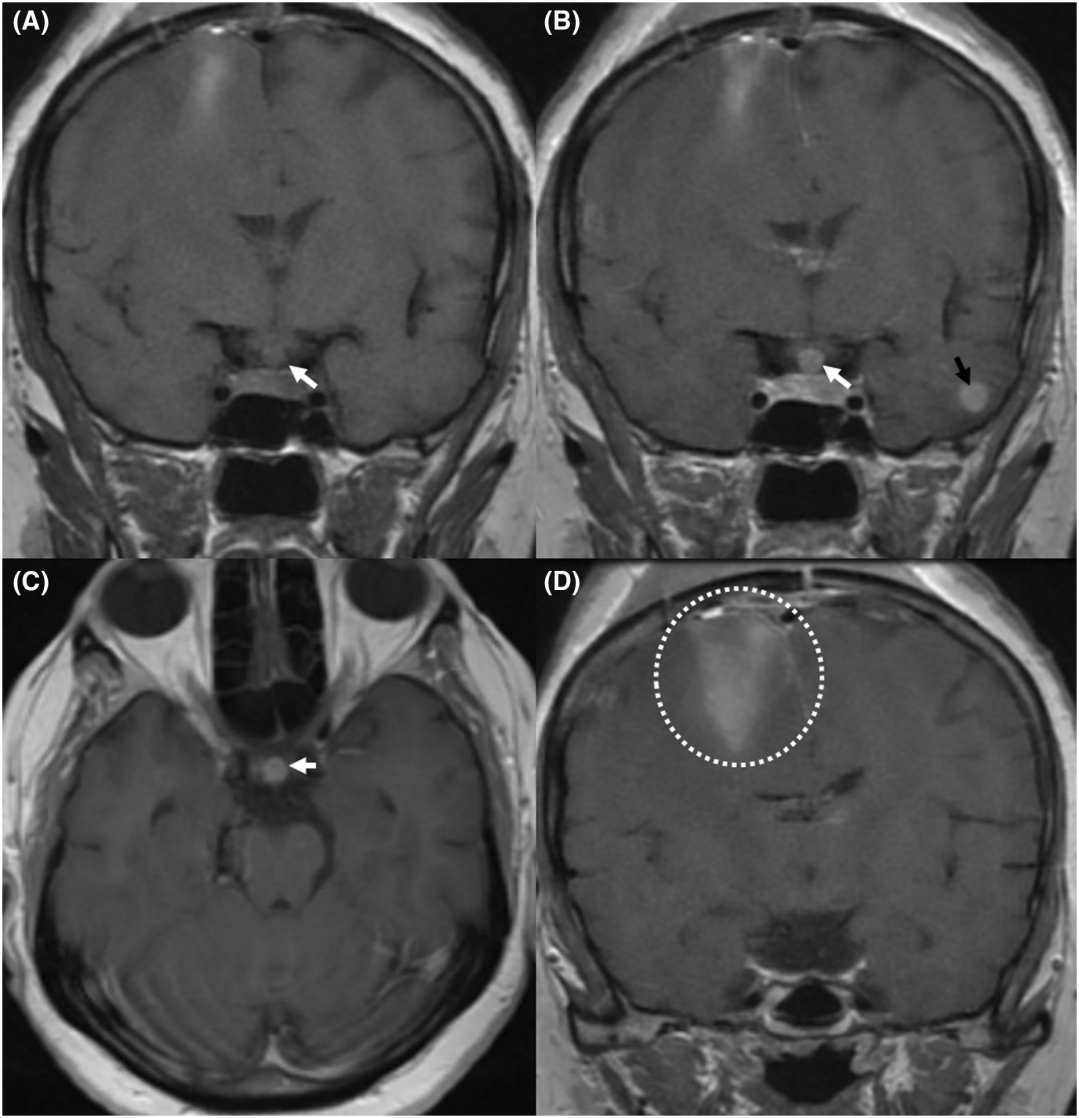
Postoperative MRI screening shows a new lesion centered on the pituitary stalk presenting as a T1 isointense round mass (white arrow in “A”) with homogeneous enhancement after gadolinium injection (white arrows in “B” and “C”). Note the left temporal lobe intra‐axial metastasis (black arrow in “B”) and the parenchymal enhancement of the operatory site (dotted circle in “D”)
